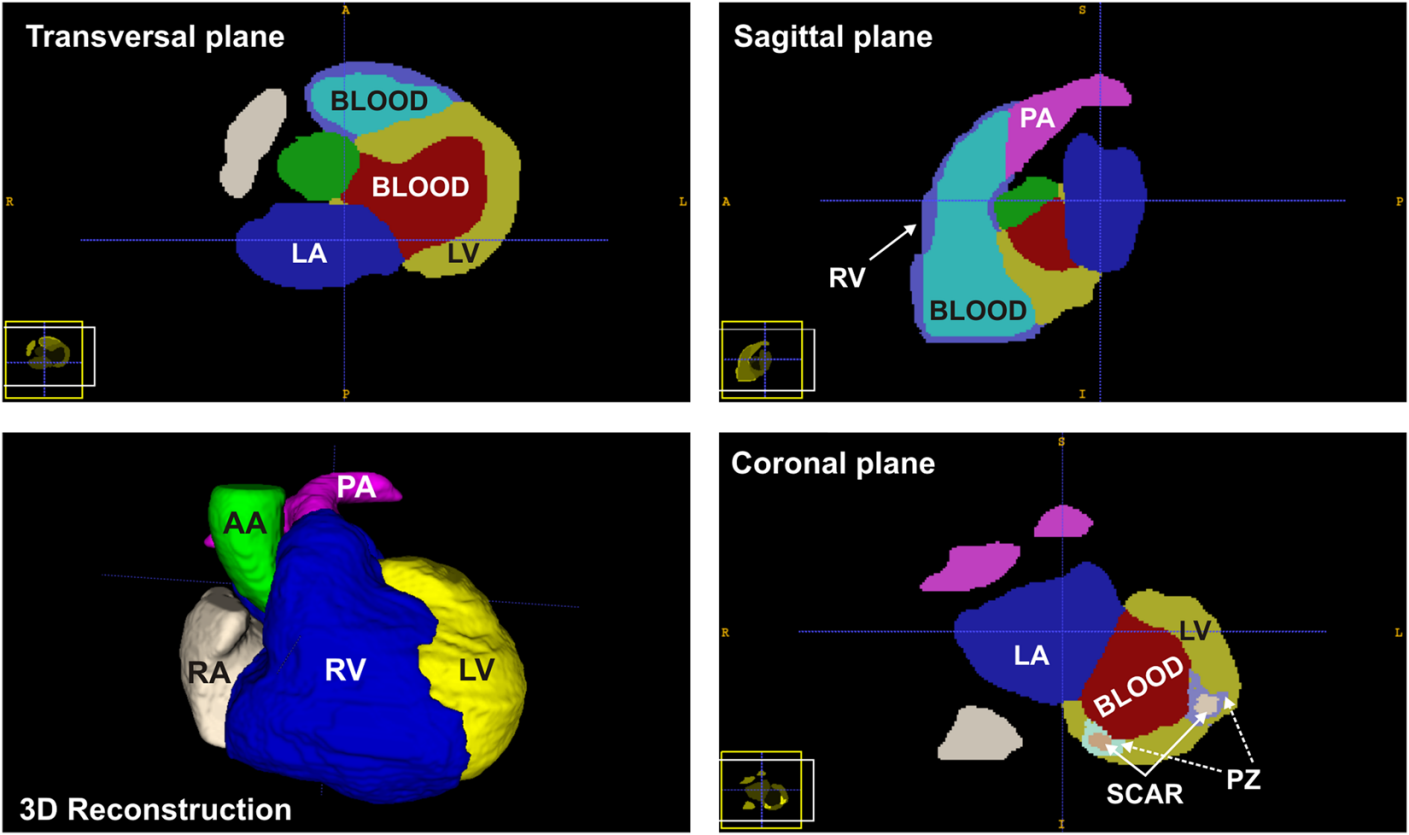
Segmented MR image. Representative 2D slices in the three main planes and 3D reconstruction are shown. Labels: right atrium (RA), left atrium (LA), right ventricle (RV), left ventricle (LV), aorta artery (AA), pulmonary artery (PA) and peri-infarct zone (PZ).
